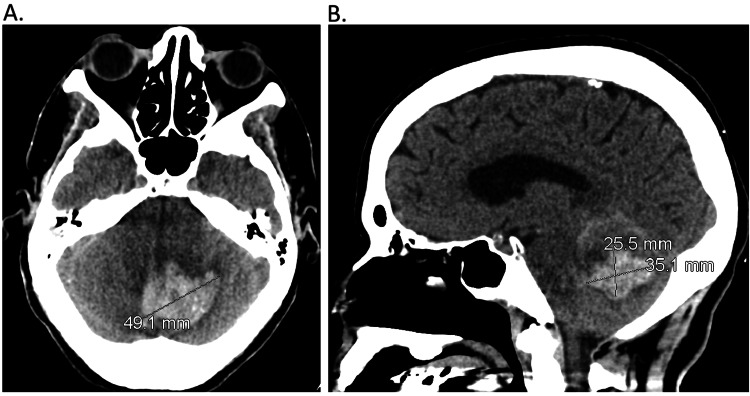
Computed tomography of the brain and head without contrast A. Transverse view showing a 49.1 mm hematoma in the cerebellum. B. Sagittal view showing a 25.5 × 35.1 mm hematoma in the cerebellum. Hemorrhage is noted and extends into the fourth ventricle with dilatation of the temporal horns suggesting probable early hydrocephalus.
